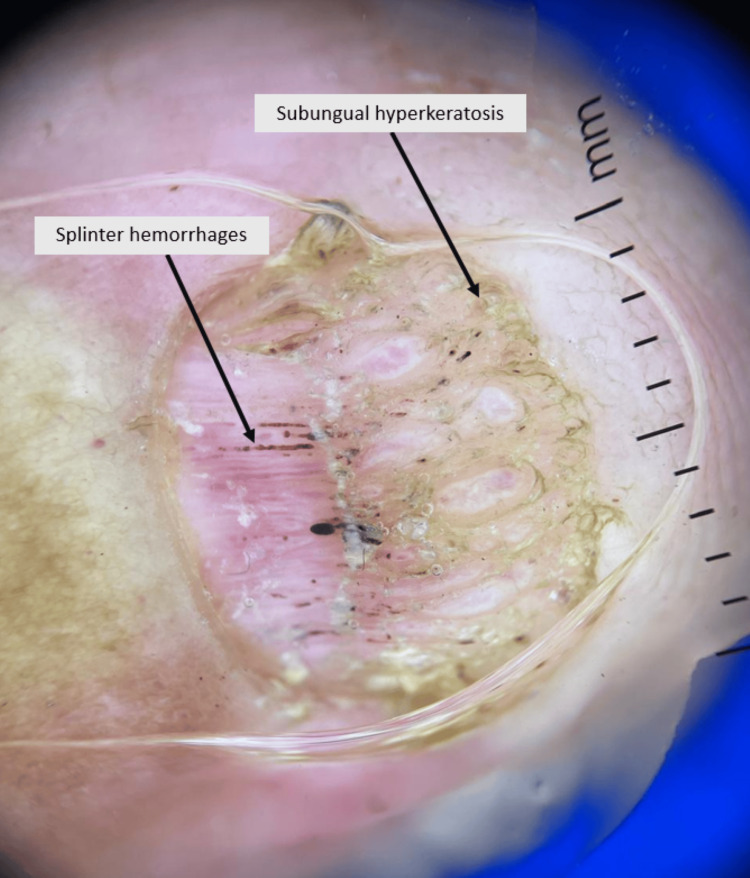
Nail-unit dermoscopy demonstrating nail plate atrophy, subungual hyperkeratosis, and splinter hemorrhages, using a polarized DermLite DL5 dermoscope with an ultrasound gel interface.
